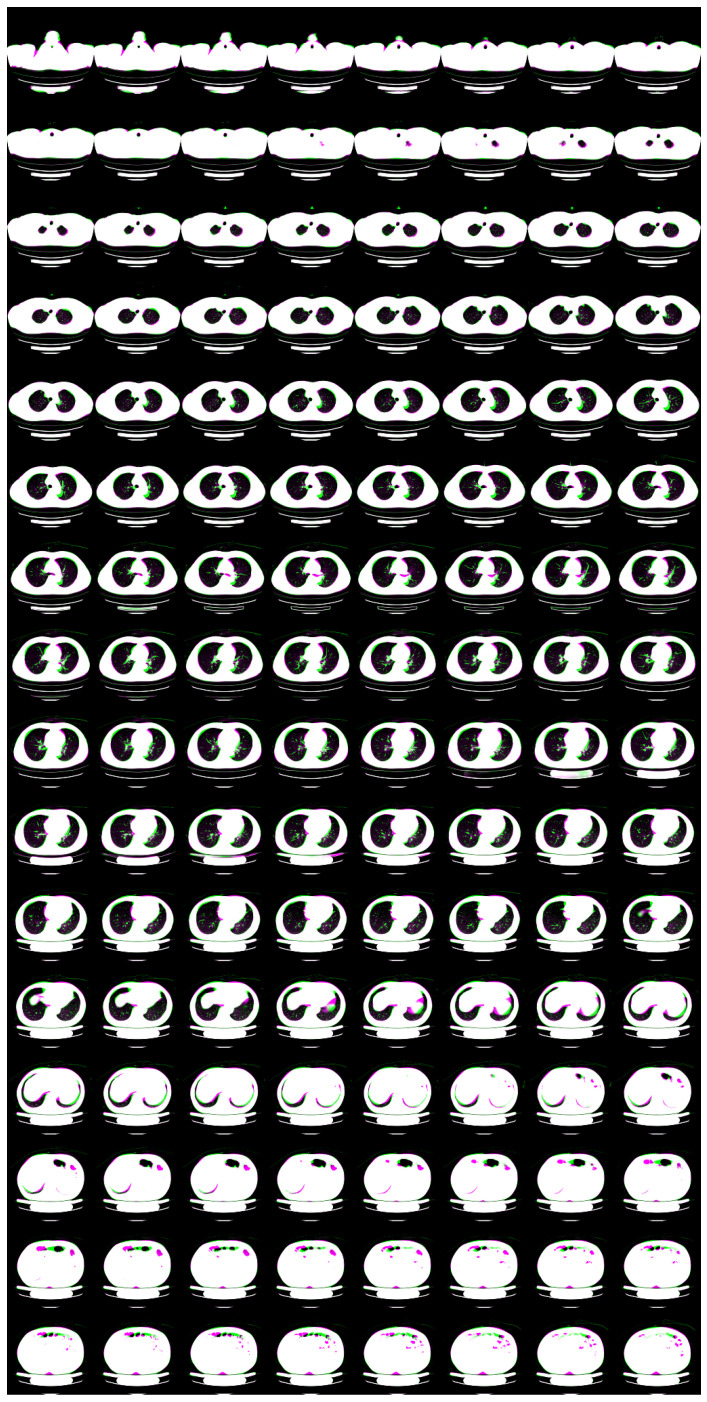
Superposition of the reconstructed 3D CT image shown in Figure 4 (magenta) and the ground-truth image (green).
